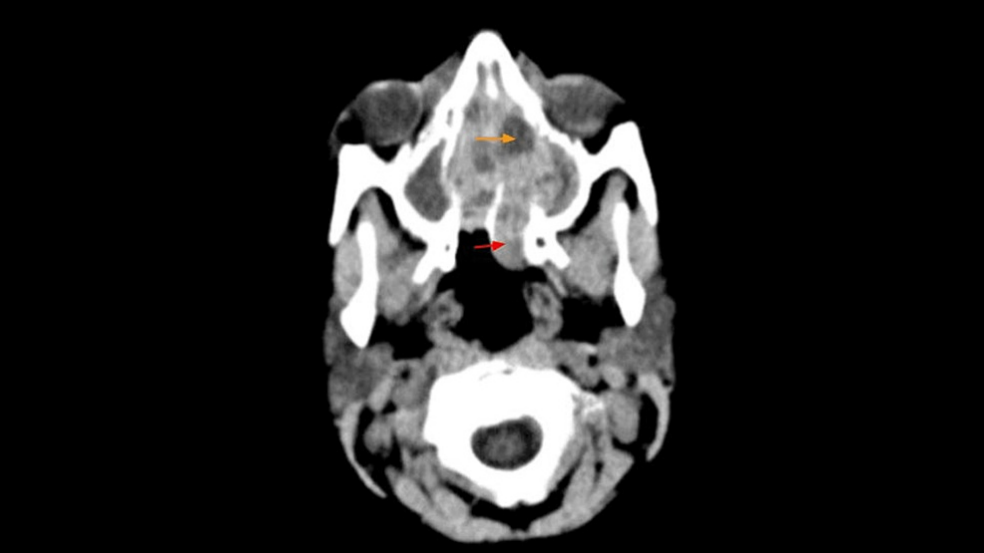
Computed tomography scan of the brain. A heterogeneous enhancement of soft tissue density seen within the nasal cavity extending through the posterior choana to the nasopharynx (red arrow) and causing some erosion in the medial wall bilaterally more in the left with extension into the left maxillary sinuses, opacified bilateral maxillary, ethmoid, and sphenoid sinuses, with right orbital media wall subperiosteal collection suggestive of abscess formation (orange arrow).
